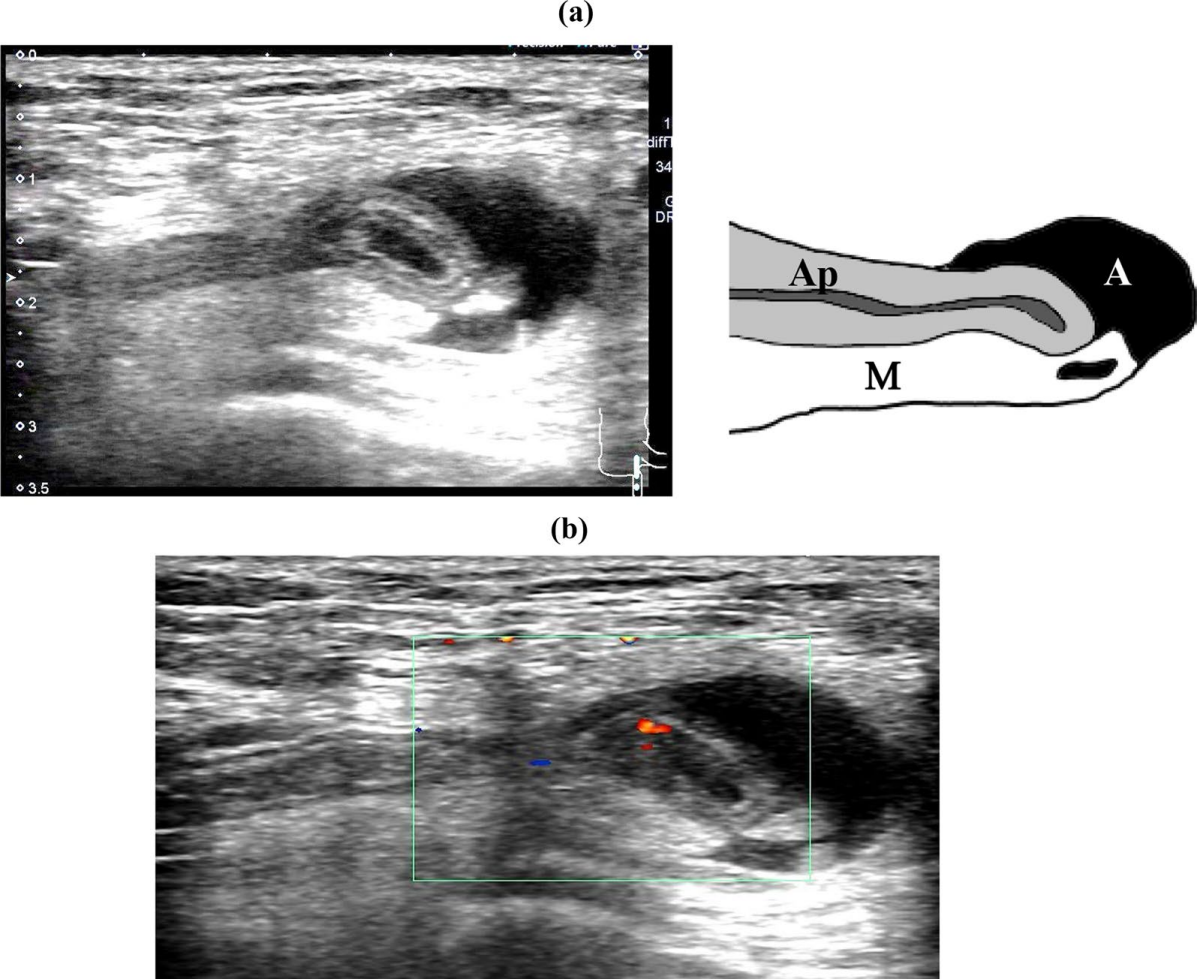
Ultrasonography (US) (Case 1). a B-mode US showing a blind-ended hyperechoic luminal structure with five layers extending from the abdominal cavity (diameter: 4 mm at the body, 6 mm at the tip), a reticular hyperechoic area, and a hypoechoic area on the medial side of the right femoral vein. They were diagnosed as appendix (Ap), mesoappendix (M), and ascites (A), respectively. b Color doppler US showing pulsatile blood flow signals in the appendiceal wall
